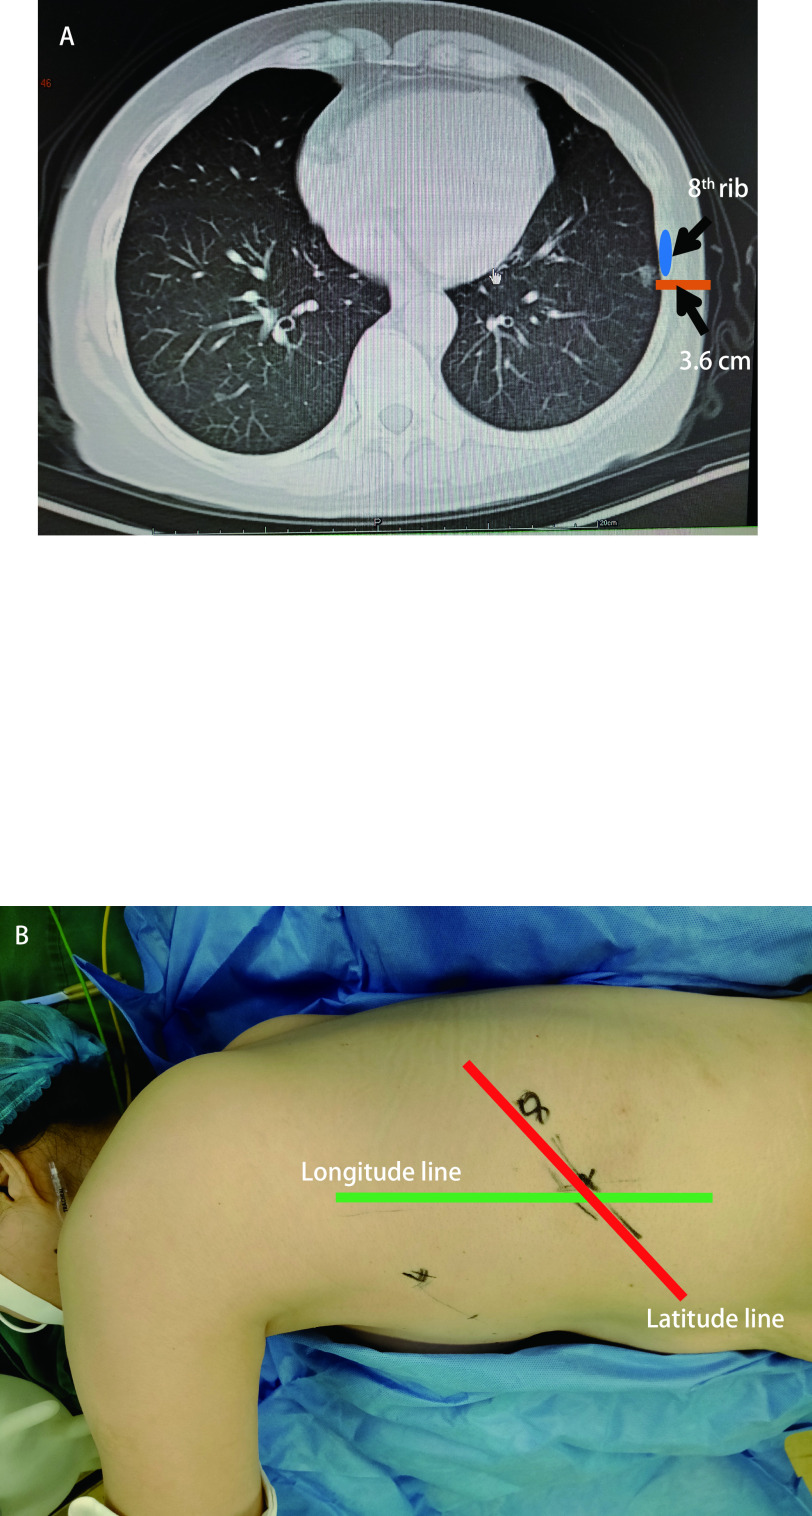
CT测量及体表经纬线确定。A：CT确定经线：侧卧位最高点垂直线；纬线：第8肋骨下缘（避开肋间血管）；穿刺深度：3.6 cm；B：体表划线：经纬线交点即为穿刺点。 CT measurement and body surface longitude and latitude line. A: CT determines the longitude line: vertical line at the highest point in lateral position; latitude line: lower edge of the 8^th^ rib (avoiding intercostal vessels); puncture depth: 3.6 cm; B: Body surface marking: the crossing point of longitude and latitude line is the puncture point. CT: computed tomography.
